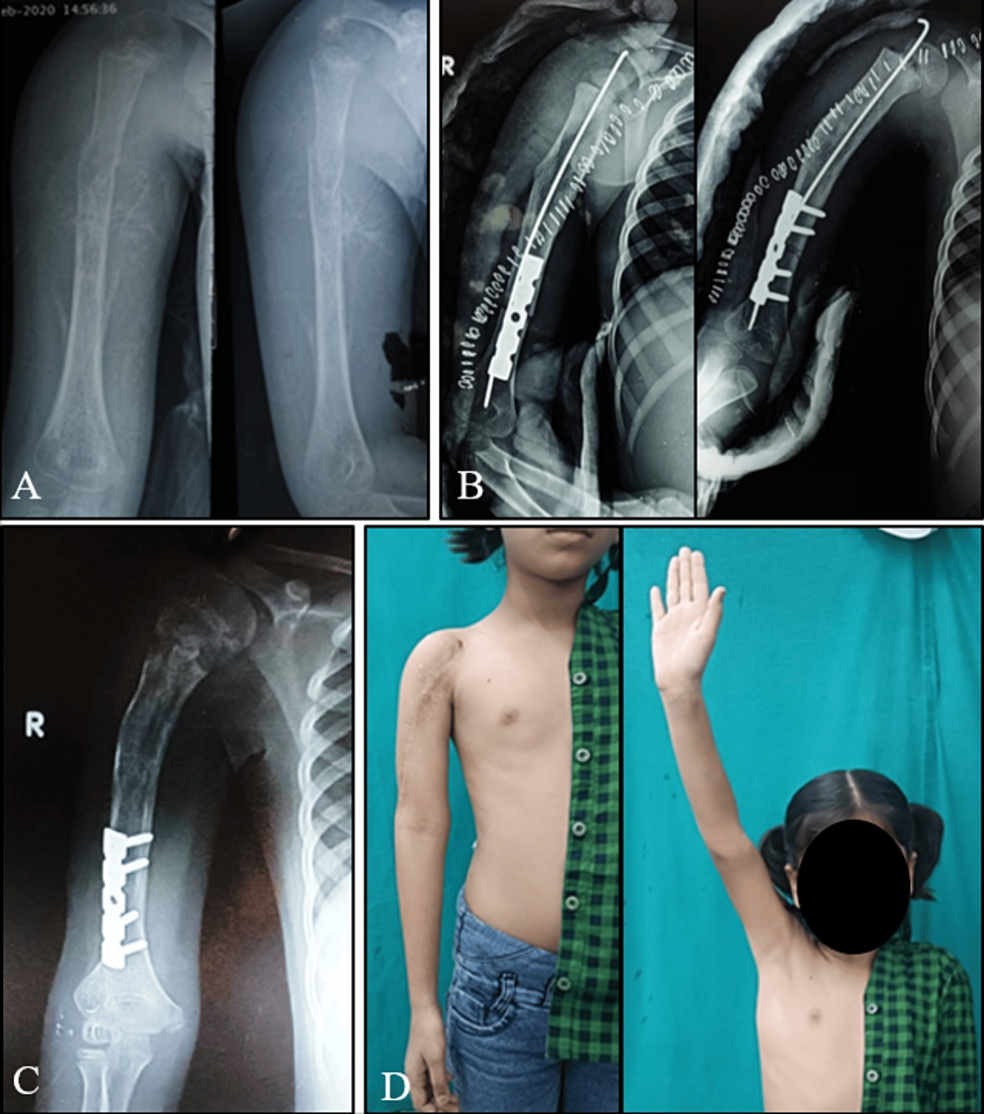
Follow-up images of a five-year-old girl with Ewings sarcoma of humerus (A) Preoperative radiograph, (B) immediate post operative radiograph, (C) follow-up radiograph at 34 months, (D) shoulder function at 34 months.
